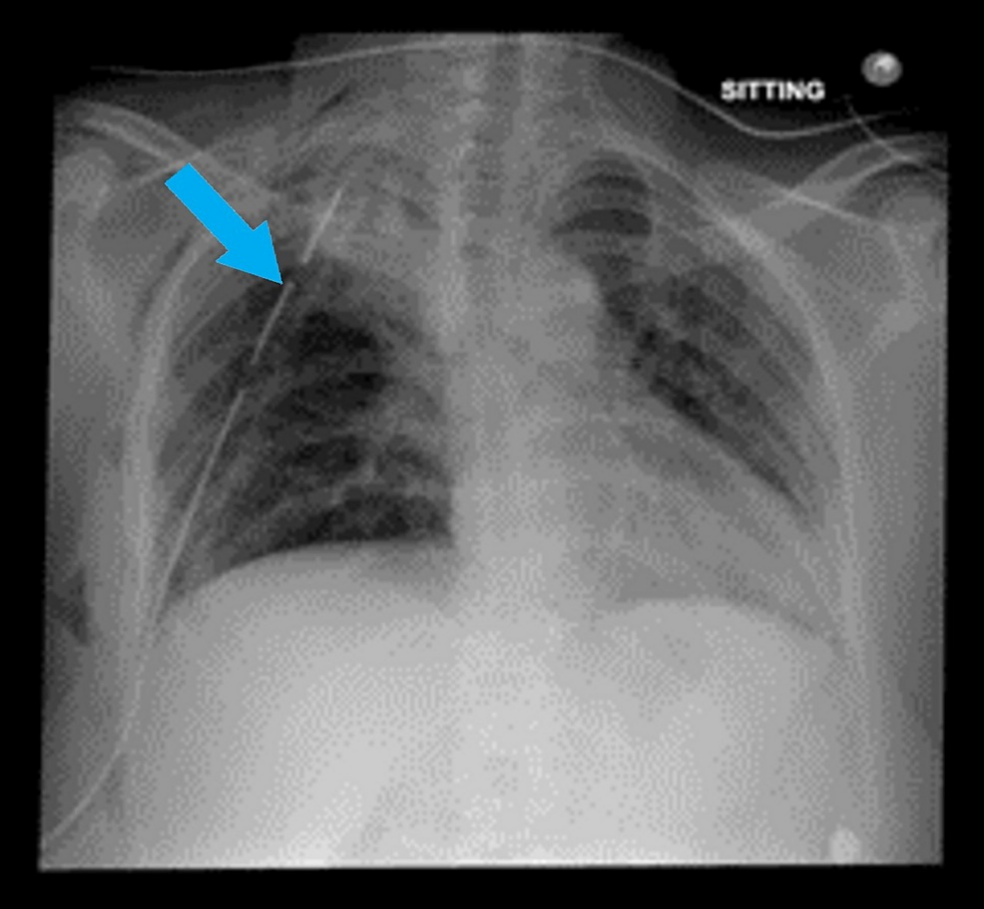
Chest x-ray on postoperative day one shows interval complete resection of the mass. A right-sided chest tube is also seen in situ.
